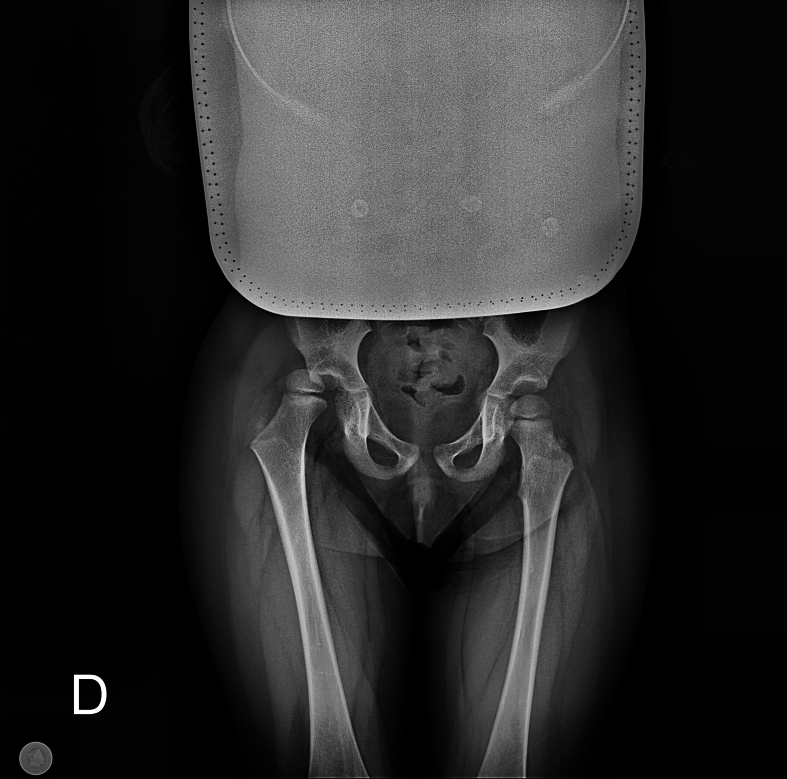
Pelvis X-ray at admission.
